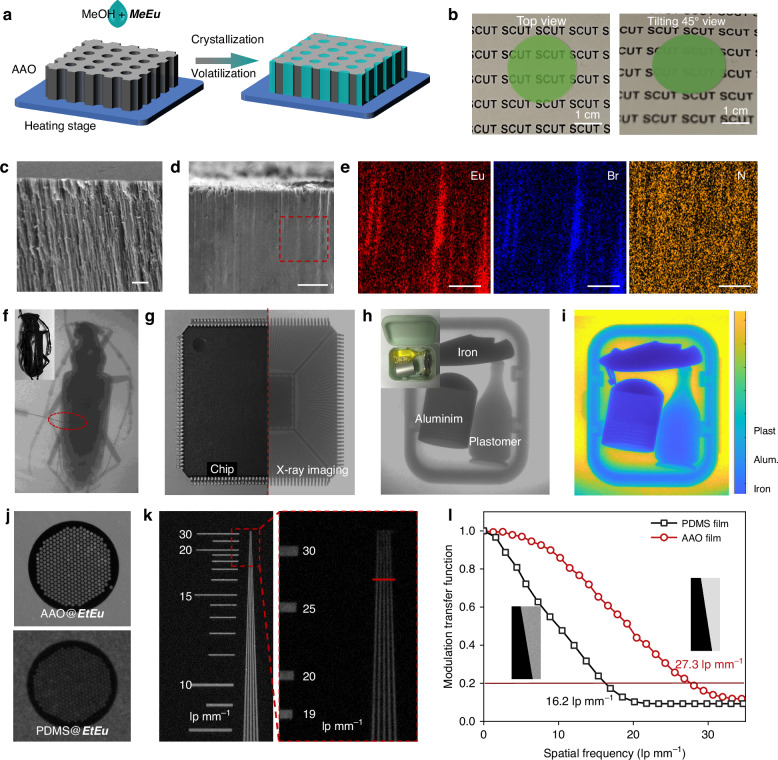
X-ray imaging of AAO@*MeEu*
